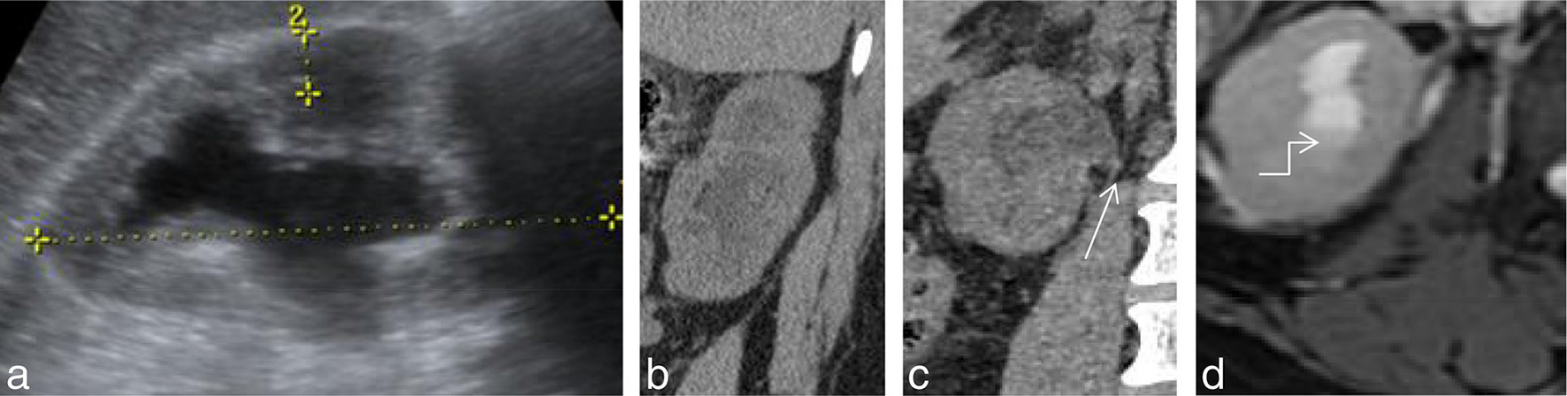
(a–d) Ultrasound image showing hydronephrosis (a) Non-contrast CT shows hydronephrosis (b) with sudden tapering of the renal pelvis (white arrow) at the pelviureteric junction. (c,d) Axial *T*_2_ weighted image showing perinephric fat stranding with debris (bent arrow) within the calyces.
